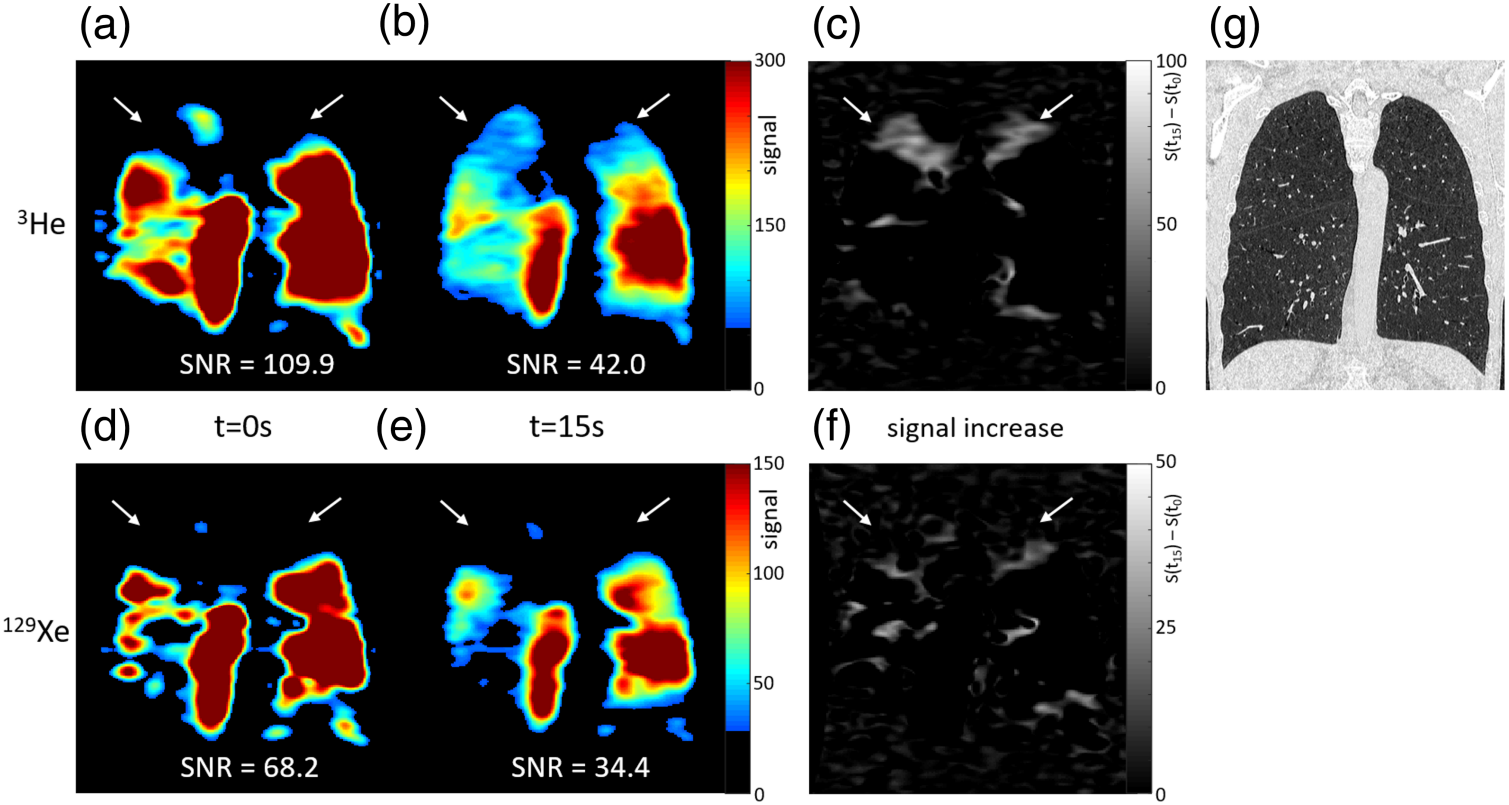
^3^He images (top) and ^129^Xe images (bottom) acquired from patient 3 during breath‐hold; (a,d) at the start of the imaging sequence, t = 0 sec, and (b,e) after 15 seconds, both shown with the same signal range. (c,f) Maps of signal increase from t = 0 sec to t = 15 sec. White arrows highlight regions of lung where ^3^He signal increased over time, but ^129^Xe signal did not. (g) CT showed severe centrilobular emphysema and hyperinflation, and all fissures appeared intact. Mean SNR over the whole lung ventilated volume for that timepoint is displayed for a, b, d, and e.
